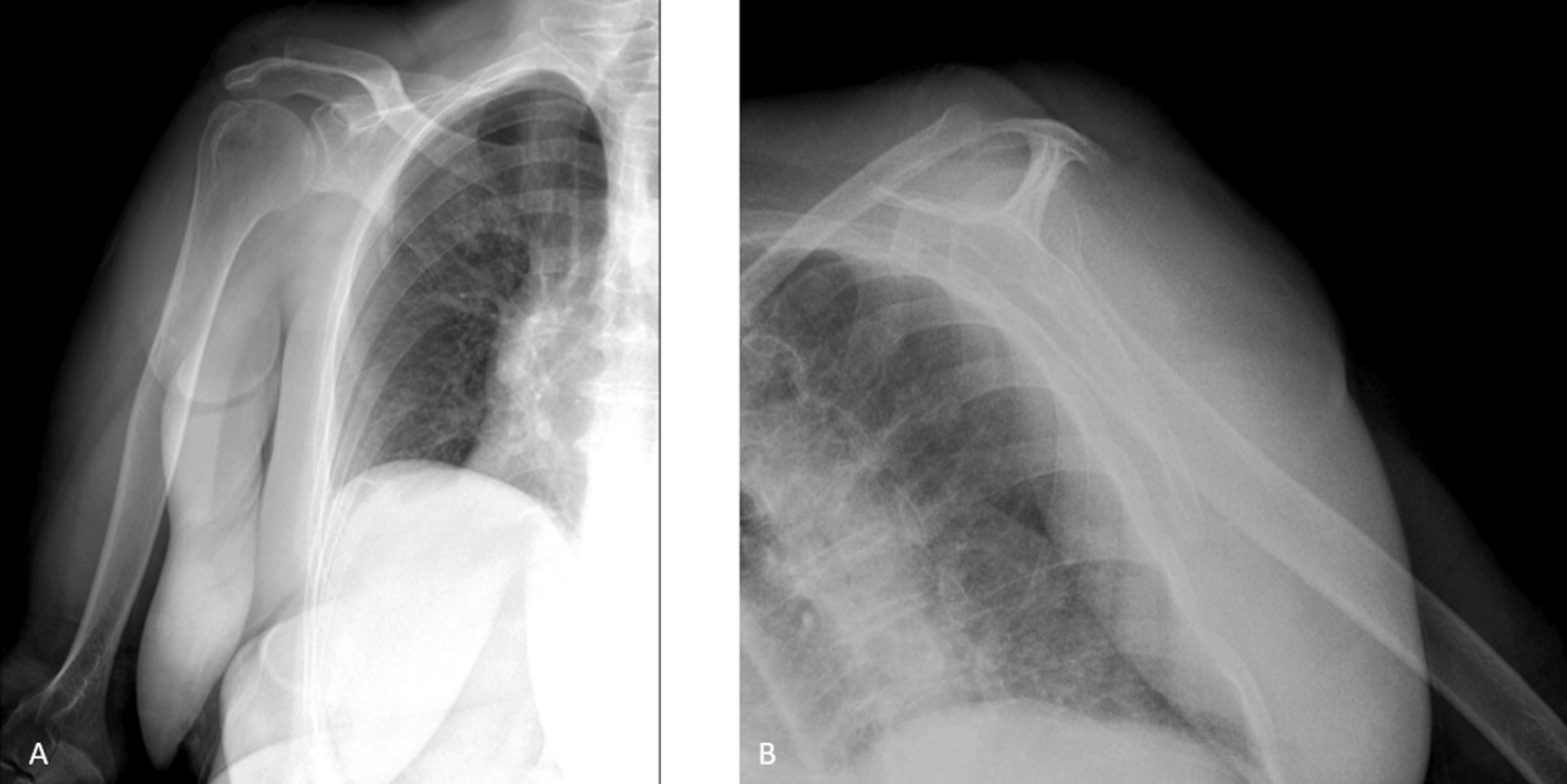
Preoperative X-ray images of the patient. (A) Anteroposterior (AP) view of the shoulder. (B) Scapular Y view of the shoulder.
